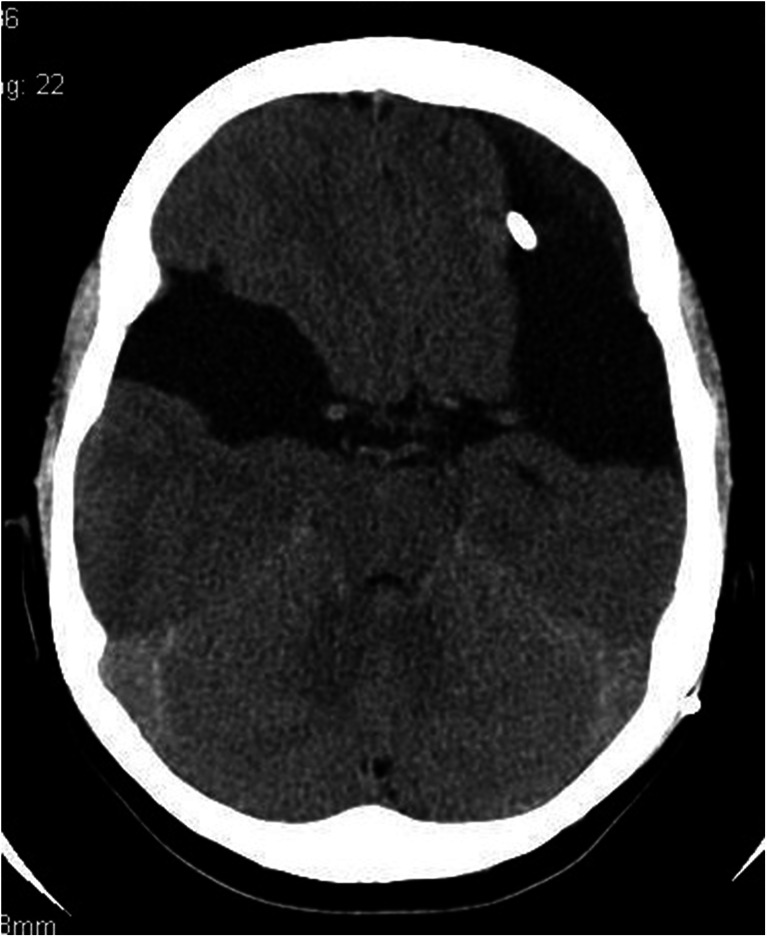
A follow-up scan, 3 years after shunt implantation: considerable regression of the bilateral arachnoid cysts in the middle cranial fossa. In the left frontal region, a narrow chronic subdural hygro-hematoma is shown, approximately 7 mm wide. Nonenhanced CT scan
